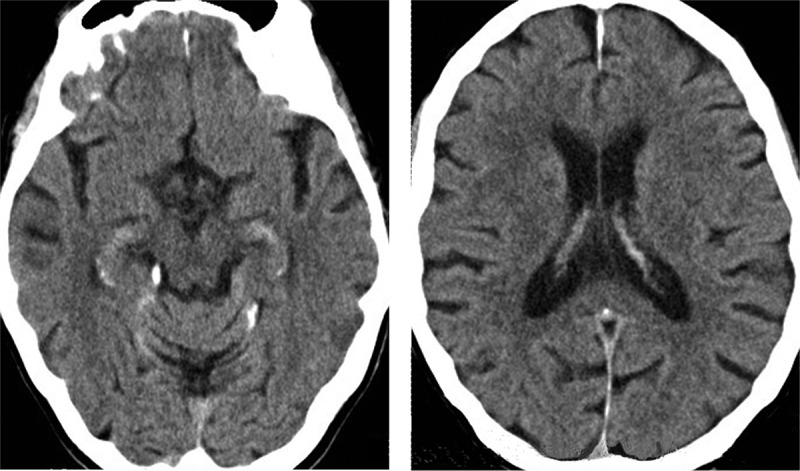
Computed tomography axial sections of the patient’s brain, at the level of the hippocampi (left panel) and inferior parietal lobes (right panel); the left hemisphere is shown on the right side of the image in both sections.
